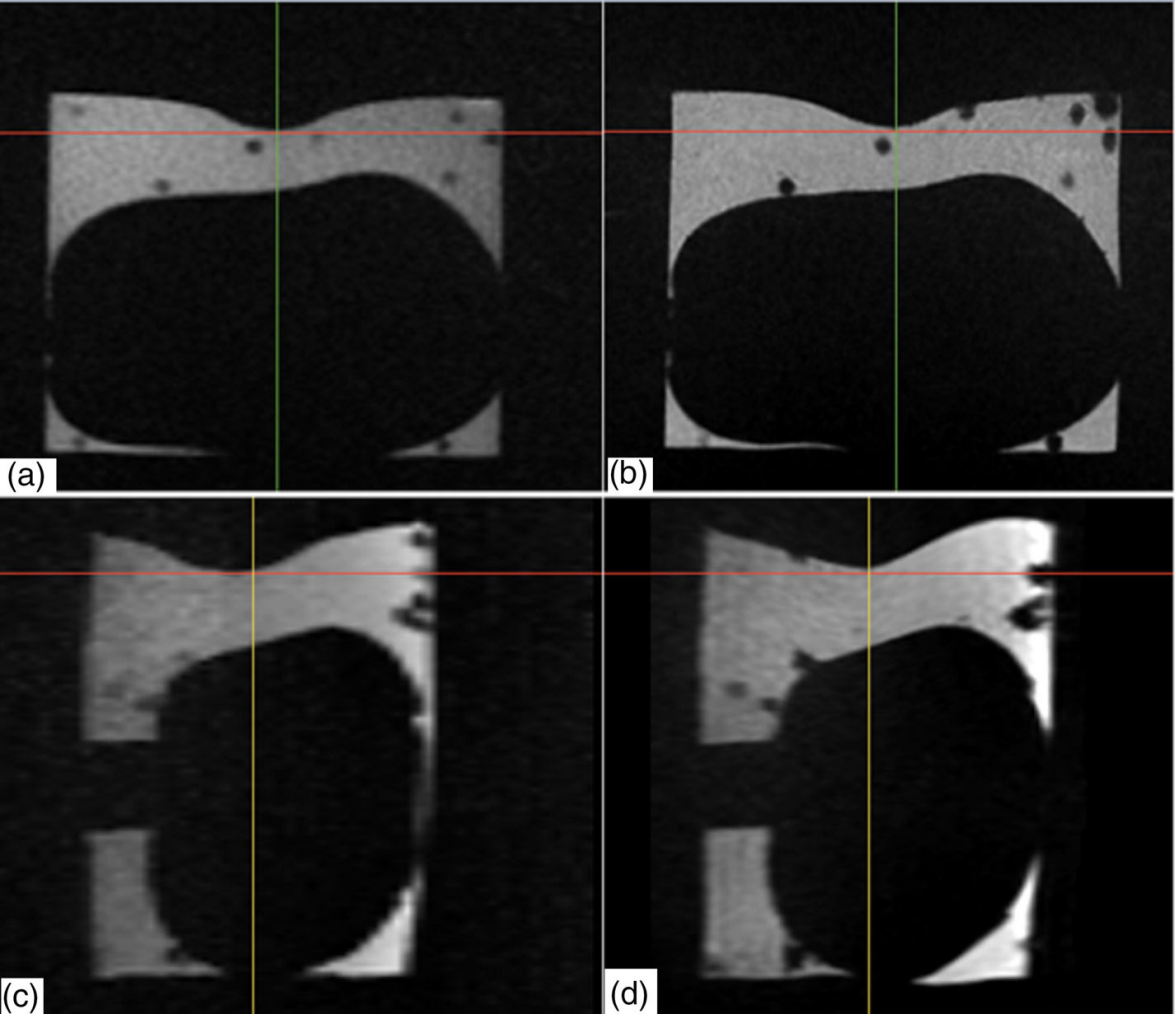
Coronal and sagittal views of the original T2‐w and the reconstructed T1‐w 4D‐magnetic resonance (MR) image at maximum displacement phase. (A) coronal view of the original T2‐w 4D‐magnetic resonance imaging (MRI) image, (B) coronal view of the reconstructed T1‐w 4D‐MR image, (C) sagittal view of the original T2‐w 4D‐MR image, and (D) sagittal view of the reconstructed T1‐w 4D‐MR image
